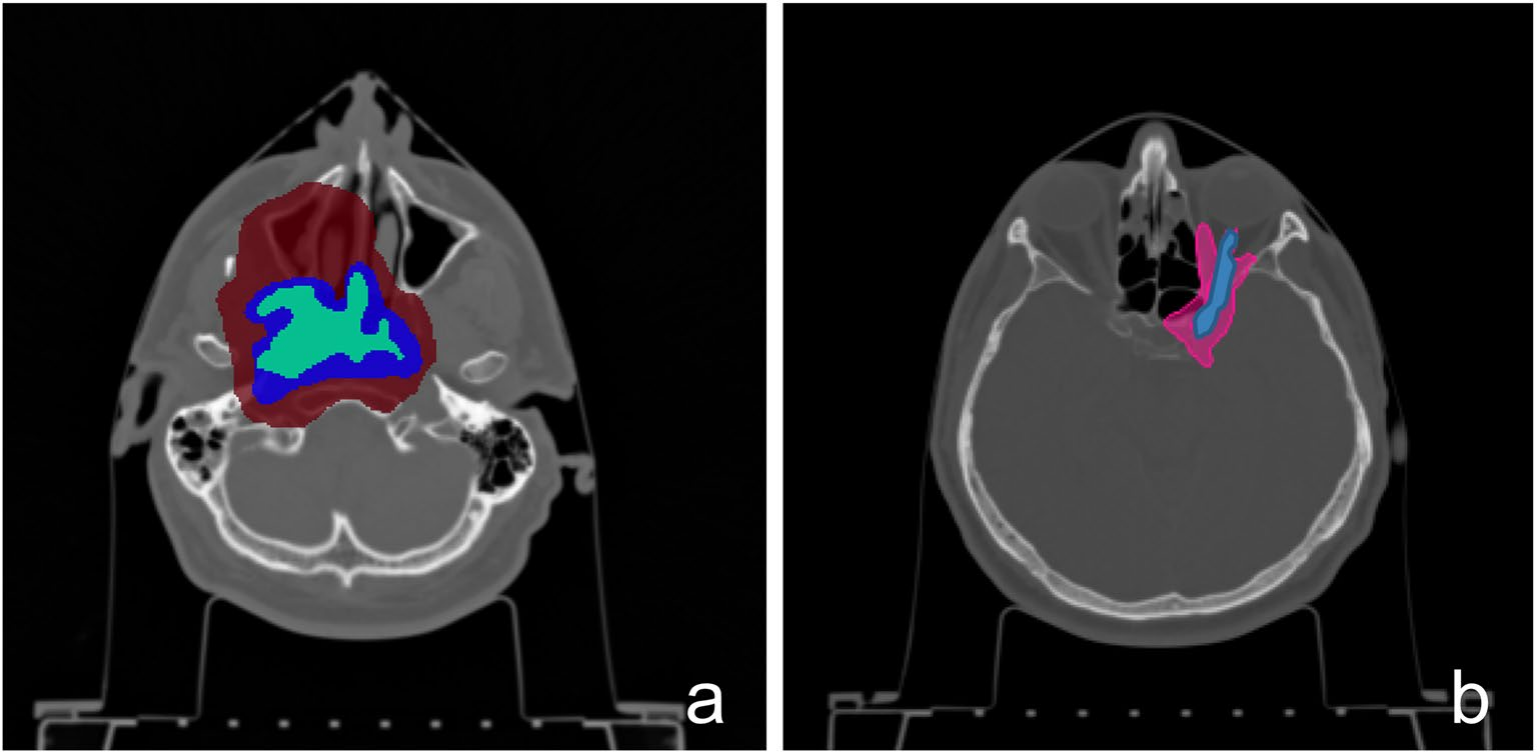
CT image of the patient: (a) with the primary tumour (in red the PTV-LD, in blue the PTV-HD and in green the GTV), and (b) with the recurrence in pink and the optic nerve in blue.
